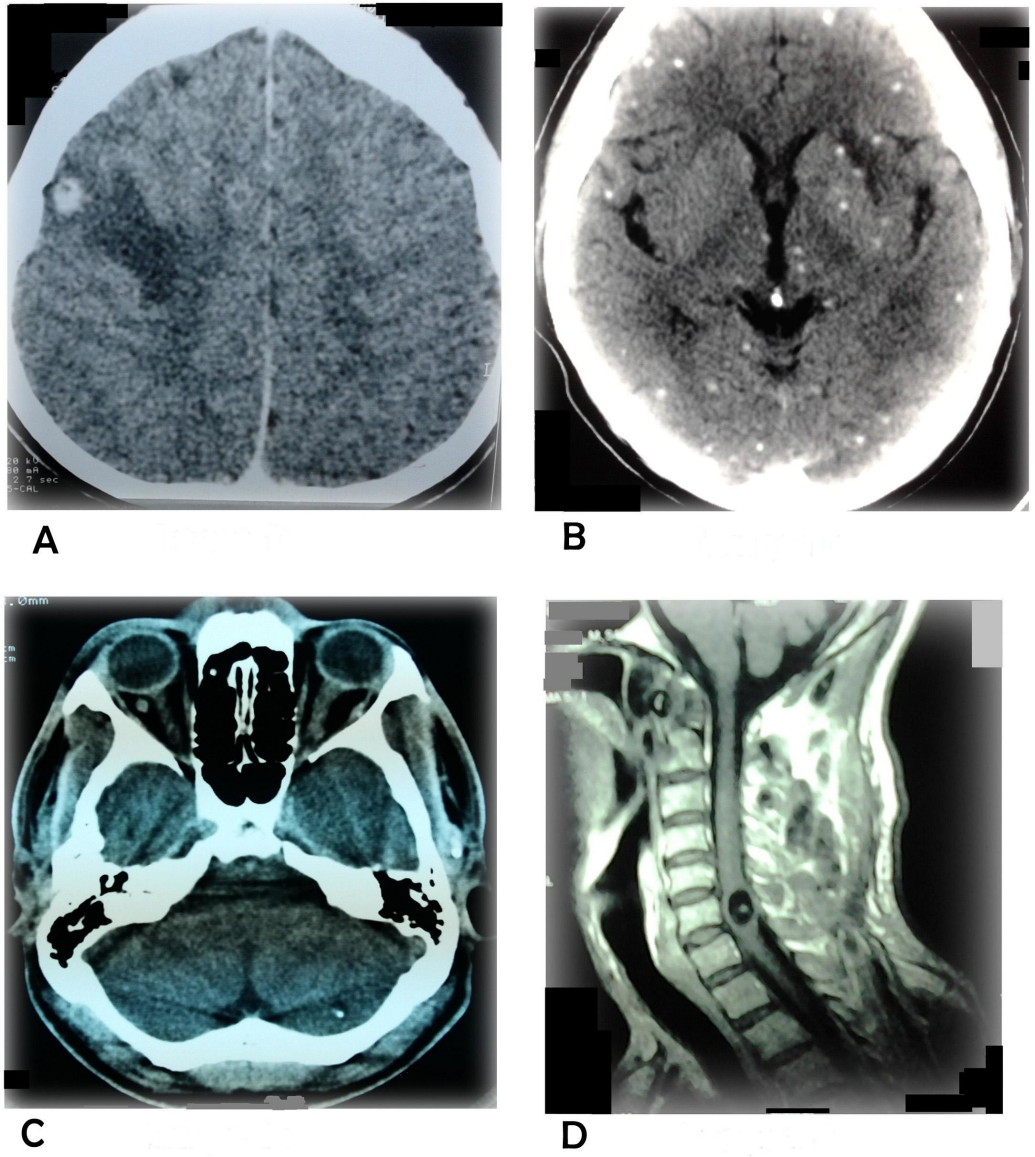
A-Single NCC with peri-lesional edema detected by CT in the right fronto-parietal area. B-Multiple NCC with starry sky pattern. C-Ocular NCC. D-Spinal NCC.
